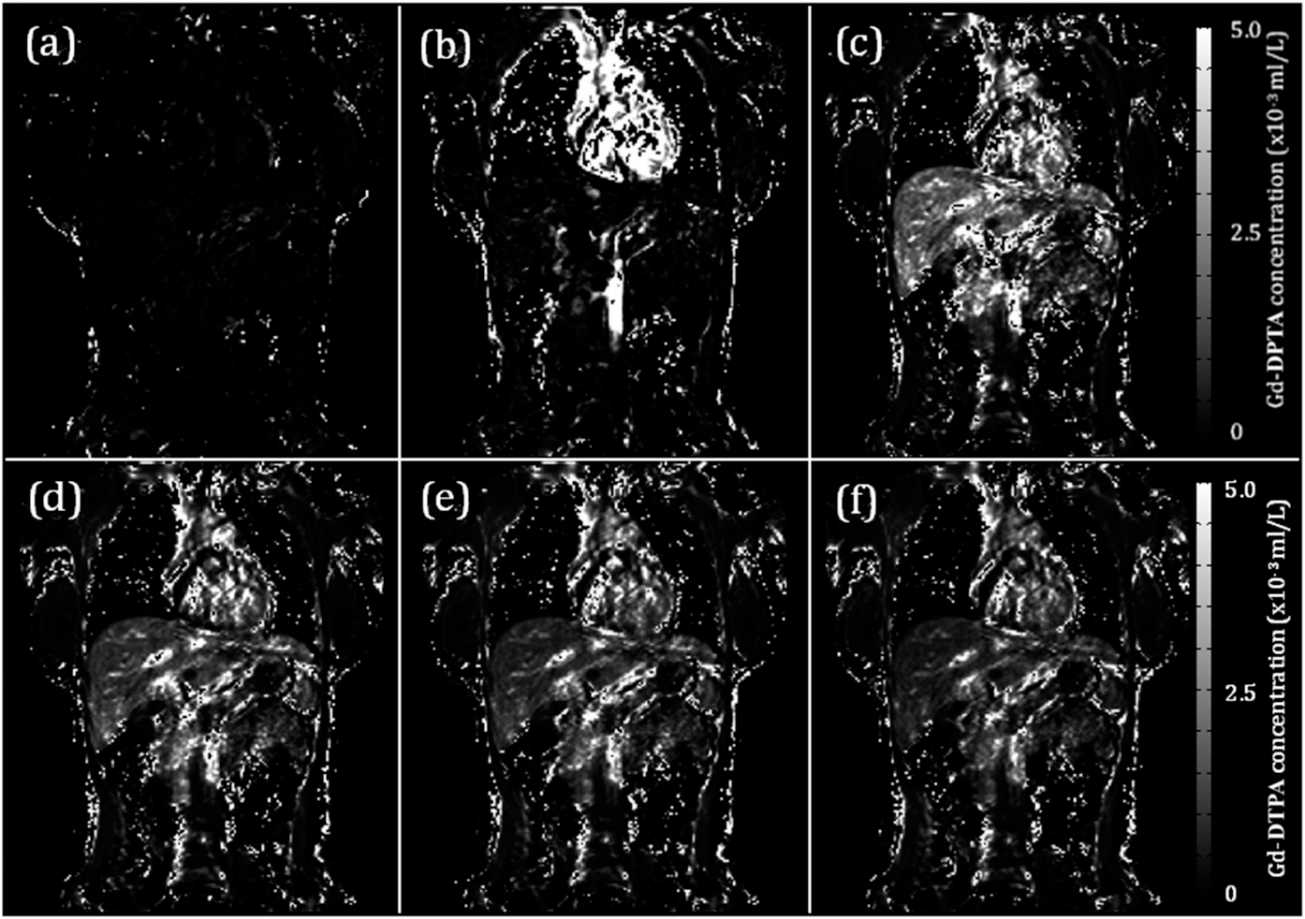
Selected gadolinium chelated with diethylene-triamine-penta-acetic acid (Gd-DTPA) concentration maps from a dynamic contrast-enhanced MRI study. Contrast agent concentration maps for a sample dataset are shown at (a) baseline, (b) 23, (c) 70, (d) 120, (e) 225 and (f) 302 s. Signal on these maps is linear to contrast agent concentration based on the scale on the right. Note the predominantly arterial phase enhancement (b), portal venous phase enhancement (c) and progressive parenchymal washout through to (f).
